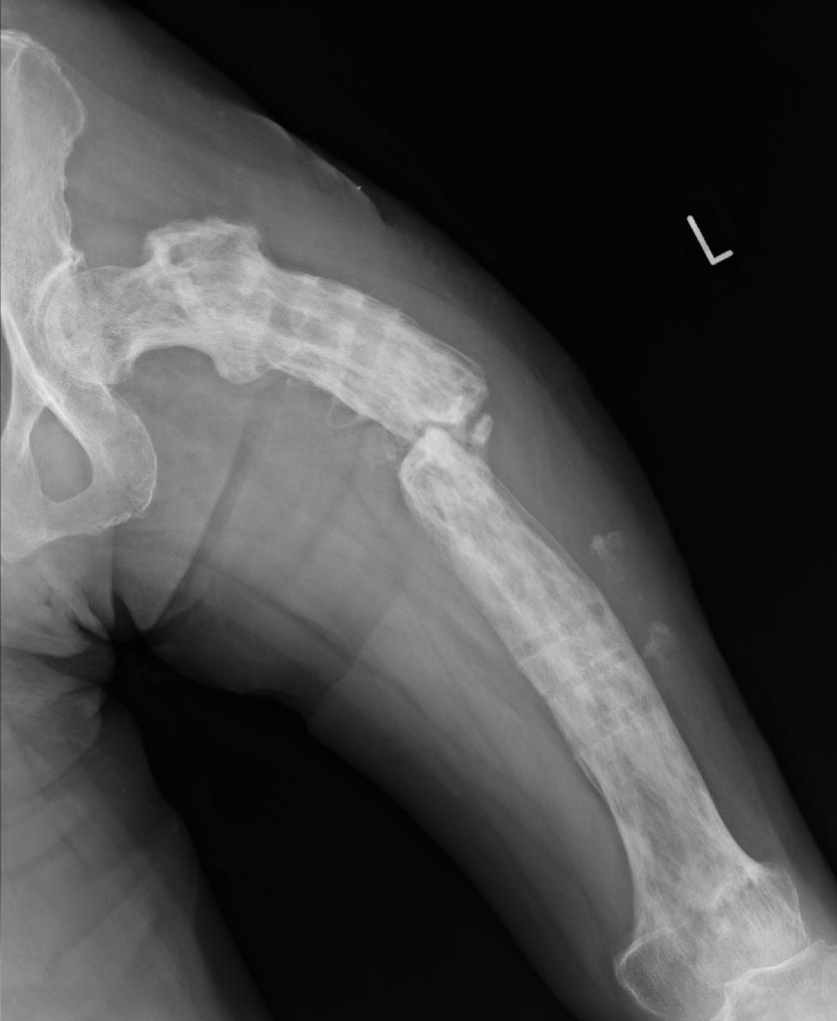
Anteroposterior radiographic view 6 month after injury shows no sign of bone healing.
